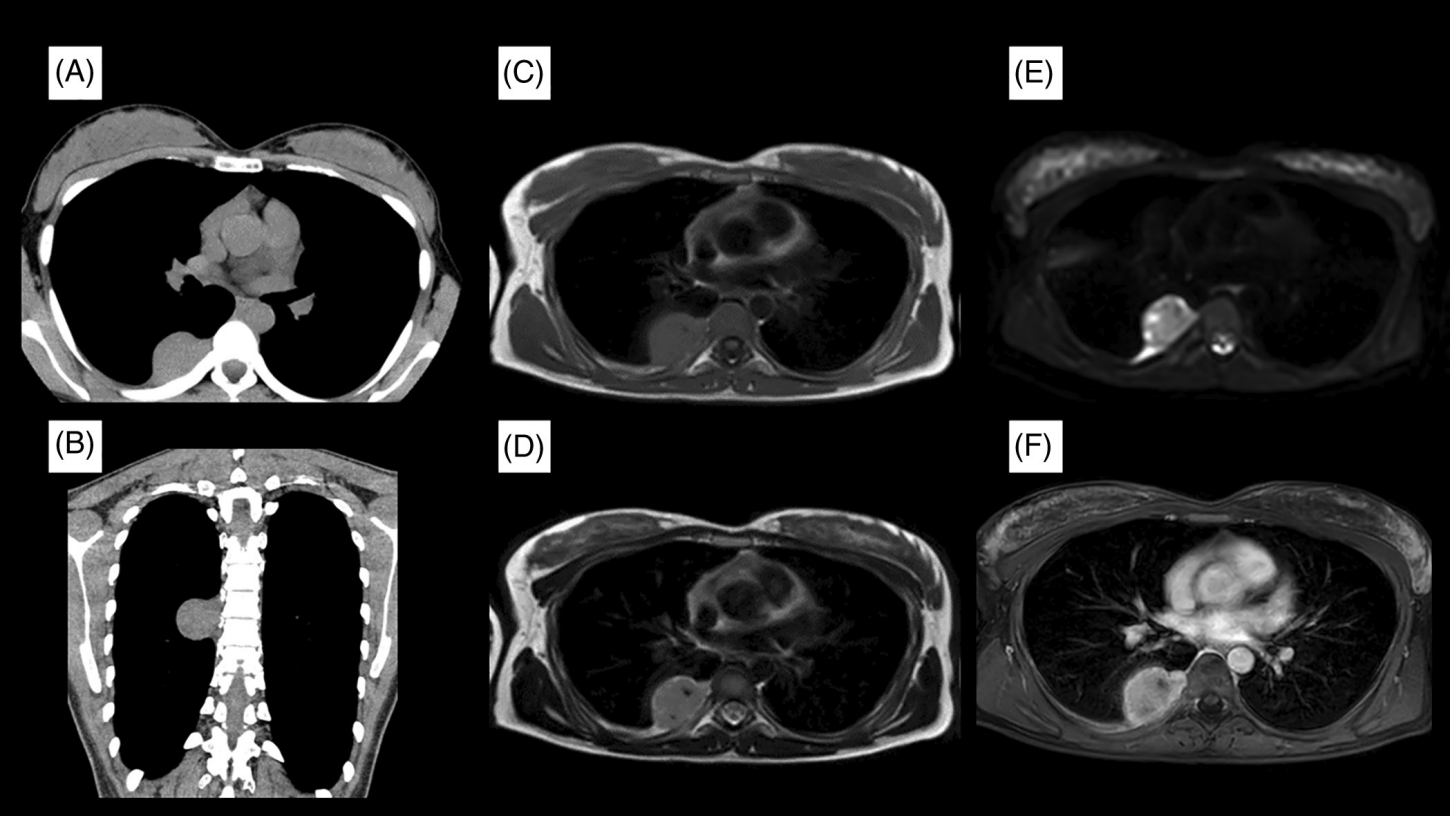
(A and B) Chest CT (mediastinal window) shows a uniform, well‐defined mass measuring approximately 40 mm in length near the vertebral body of the seventh thoracic vertebra. (C) Chest MRI (T1WI) shows a mass adjacent to the pleura with signal intensity comparable to muscle. (D) Chest MRI (T2WI) shows the edges were high‐signal. (E) Chest MRI (diffusion weighted images) shows diffusion restriction around the limbus of the mass. (F) Chest MRI (Contrast‐enhanced T1WI) shows strong, homogeneous enhancement of the mass.
